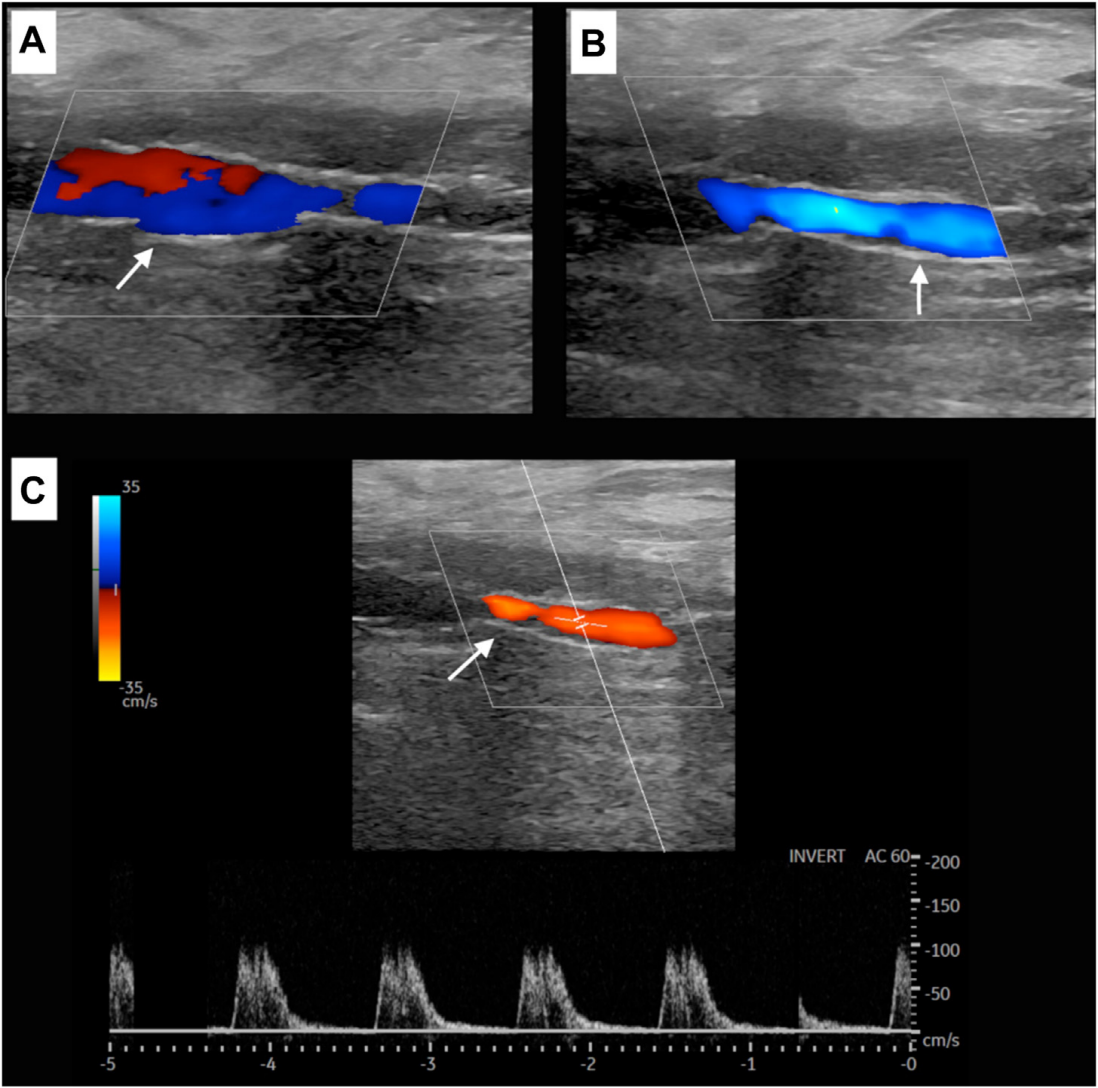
At 6 months after intervention, color Doppler ultrasound scans demonstrating regular stent patency (*white arrow*) in common femoral artery (CFA; A) and deep femoral artery (DFA; B), with no signs of stent fracture or intraluminal hyperplasia. The peak systolic velocity flow within the stent was ∼100 cm/s (C).
